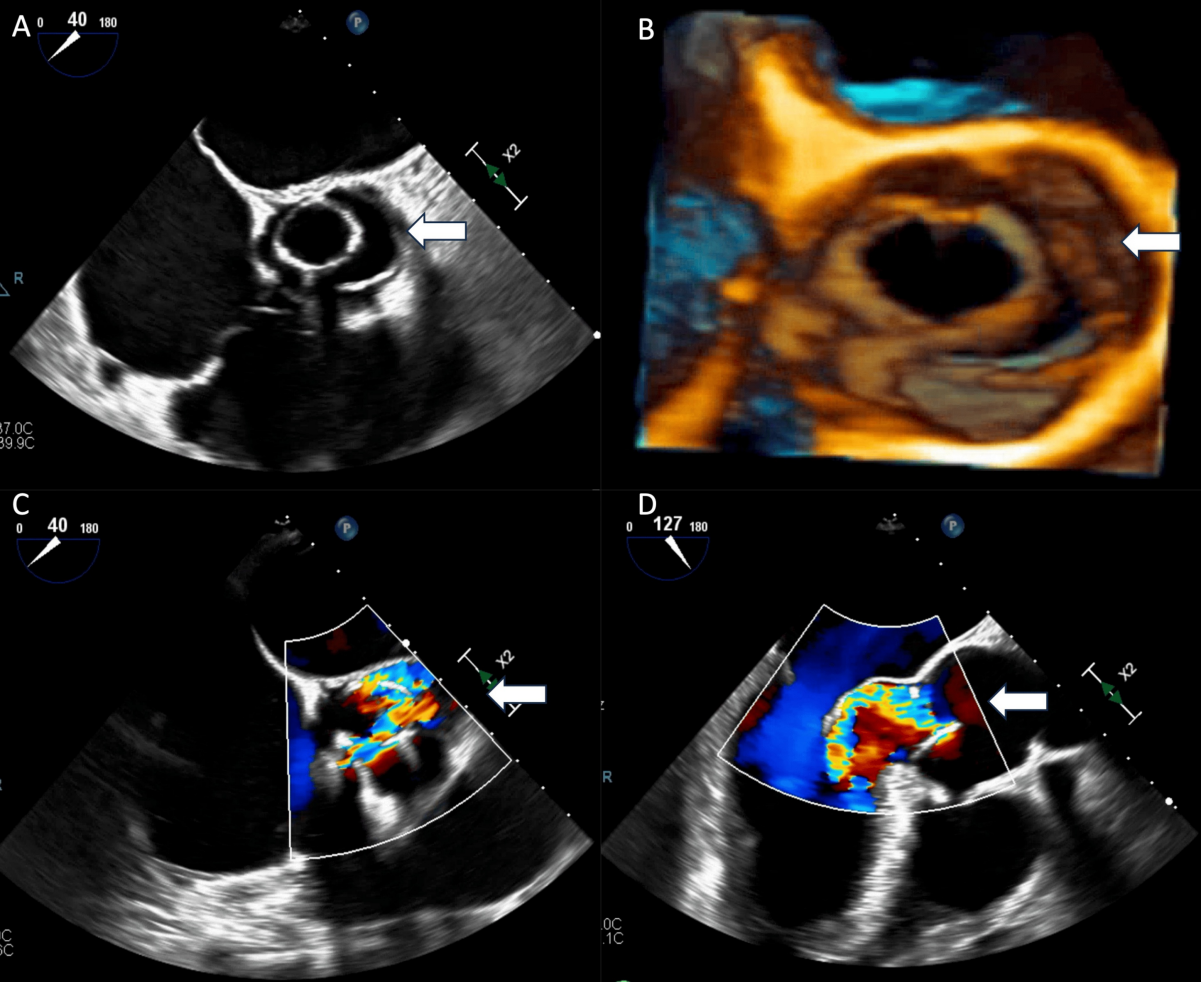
Transesophageal echocardiogram on postoperative five months (A, B) Aortic annular ring not attached to the anterior aspect of the aortic root (white arrow). (C, D) Severe aortic insufficiency detected with eccentric jet (white arrow)
